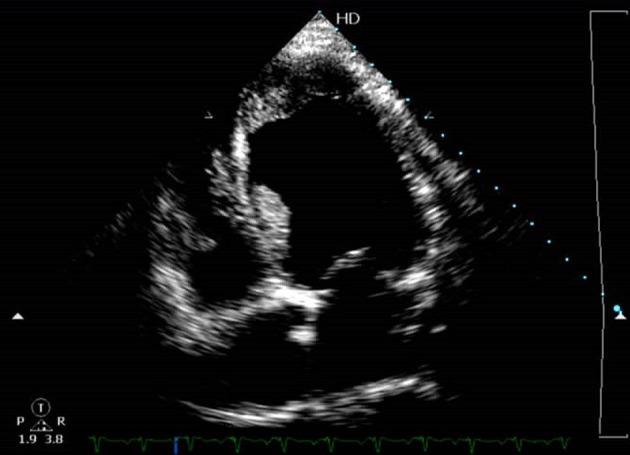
Echocardiography (apical four-chamber view) showing dilated chambers, with layered left ventricular thrombus.
